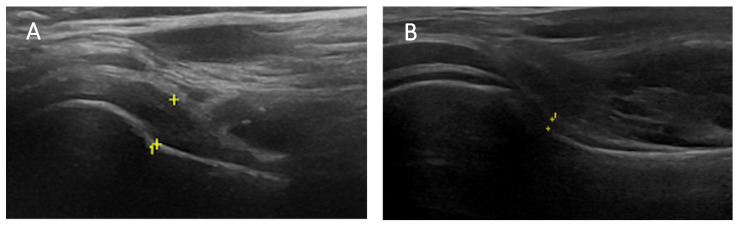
AP thickness is evaluated by positioning the probe longitudinally along the mid-axillary line, measuring the distance between the cortical line of the humeral neck to the outer margin of the glenohumeral joint. On the left (A) is a thickened AP (5.1 mm) of the affected shoulder. On the right (B) is a normal AP (1.1 mm) of the contralateral shoulder. Symbol (+) represents measurement landmarks in ultrasound images.
